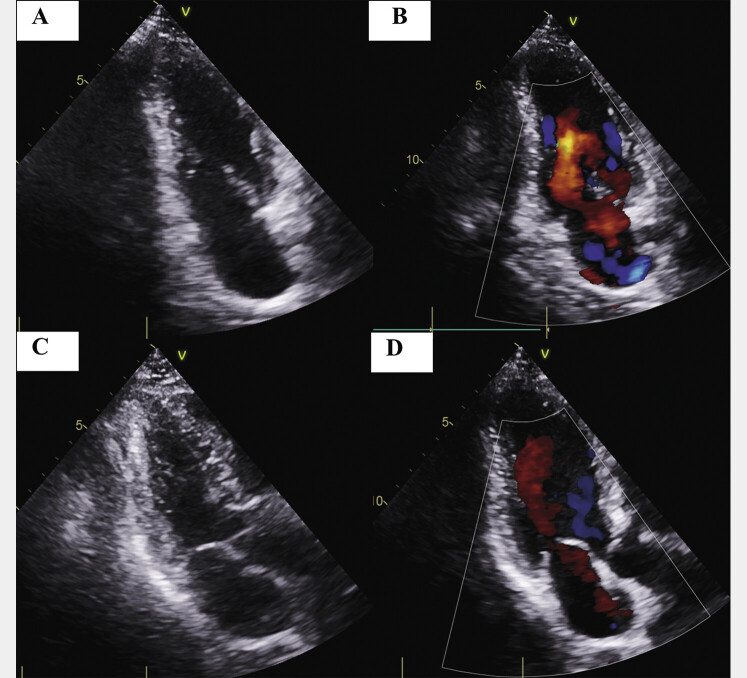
Transthoracic echocardiogram did not detect any occupying lesion from the apical two-chamber view (
